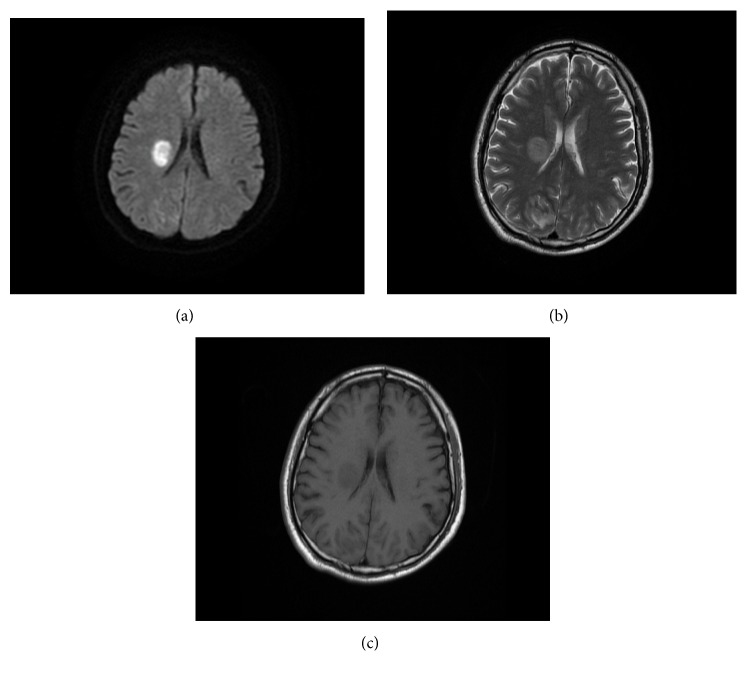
Brain MRI performed on the third day revealed (a) high signal intensity on DW image and (b, c) well-defined rounded cystic lesion on the T2-weighted and T1-weighted images involving in the right corona radiata and basal ganglia, compressing the right lateral ventricle with midline shift to left, suggestive of an early stage of brain abscess.
